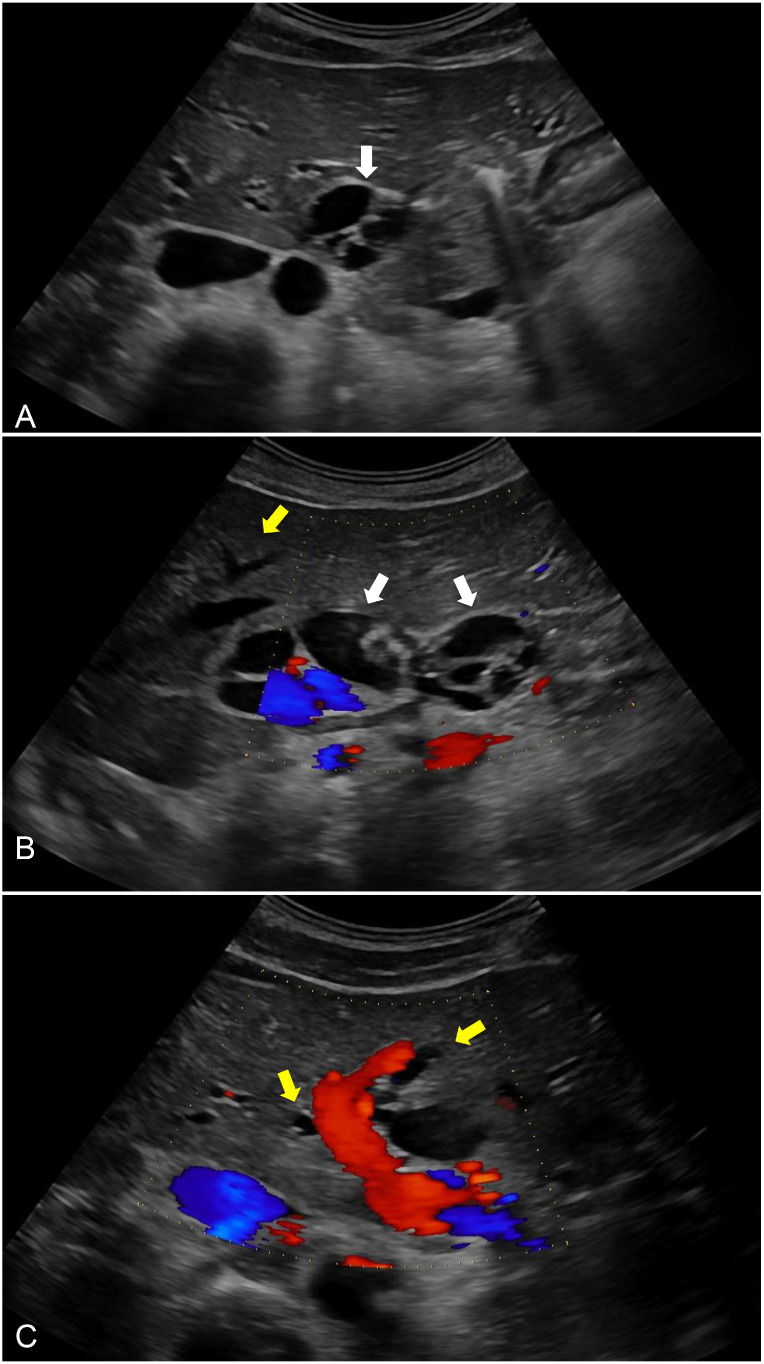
Ultrasound of the abdomen: (A) Transverse decubitus grayscale ultrasound of the gallbladder demonstrates a multiseptated cystic lesion (white arrow) within the gallbladder fossa. (B) Sagittal decubitus ultrasound of the gallbladder with color Doppler shows no internal vascularity within the lesion (white arrows) and associated intrahepatic bile duct dilation (yellow arrow). (C) Color Doppler evaluation of the main portal vein demonstrates preserved flow. There is intrahepatic and extrahepatic bile duct dilation (yellow arrows).
